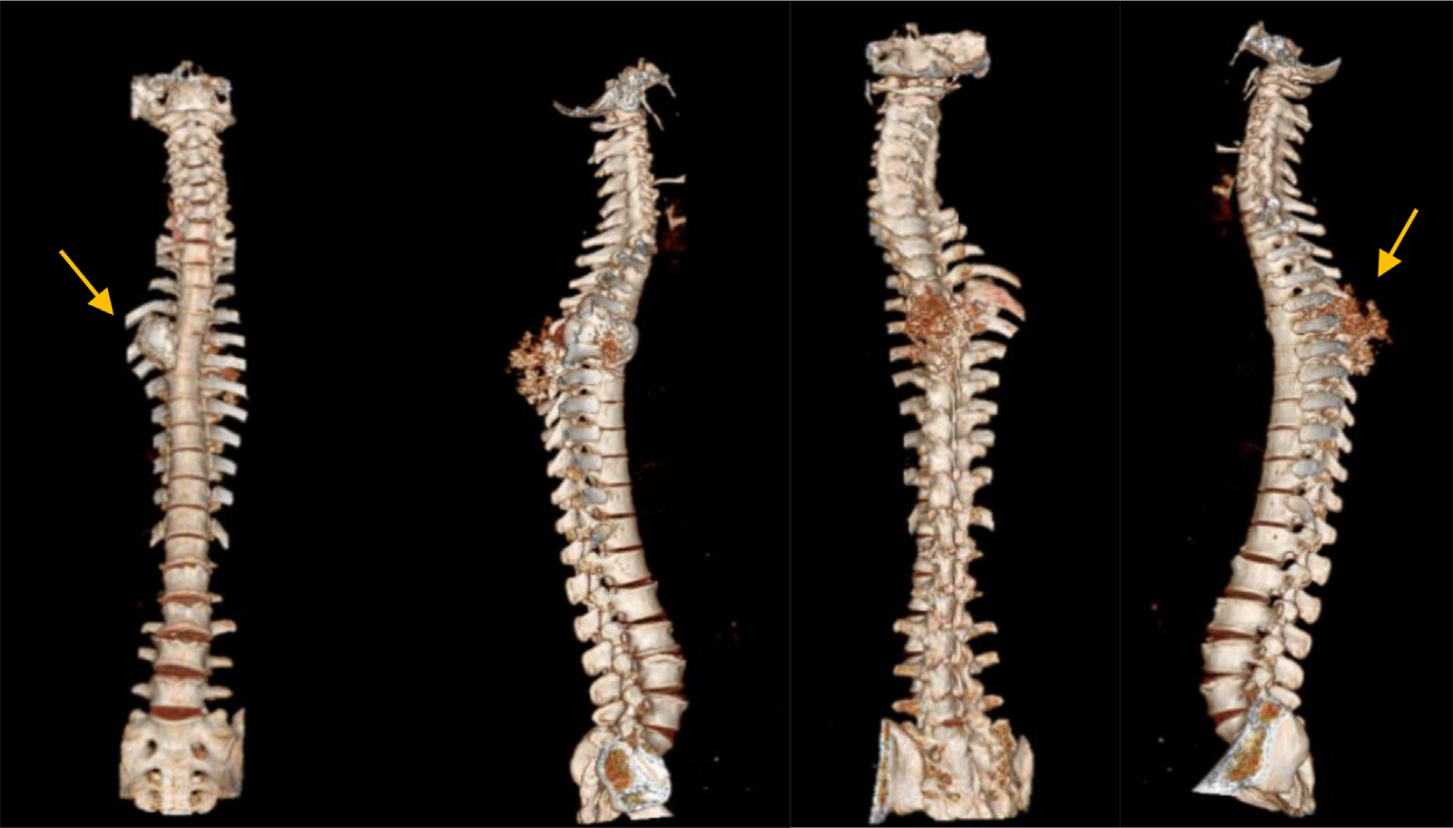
Computerized tomography scan of column vertebrae region with 3D construction (CT- Scan Hitachi Scenaria 2017). A mass located in the right posterolateral aspect of the thoracic vertebrae T5-T6 (yellow arrow).
